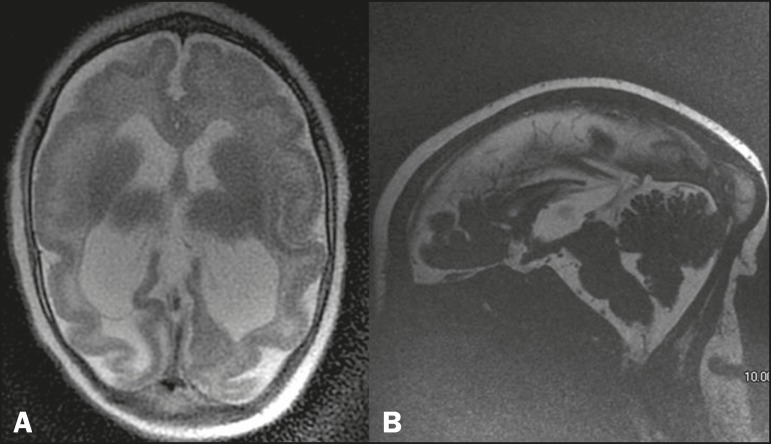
MRI: axial T2-weighted sequence (A) showing hypogenesis of the corpus
